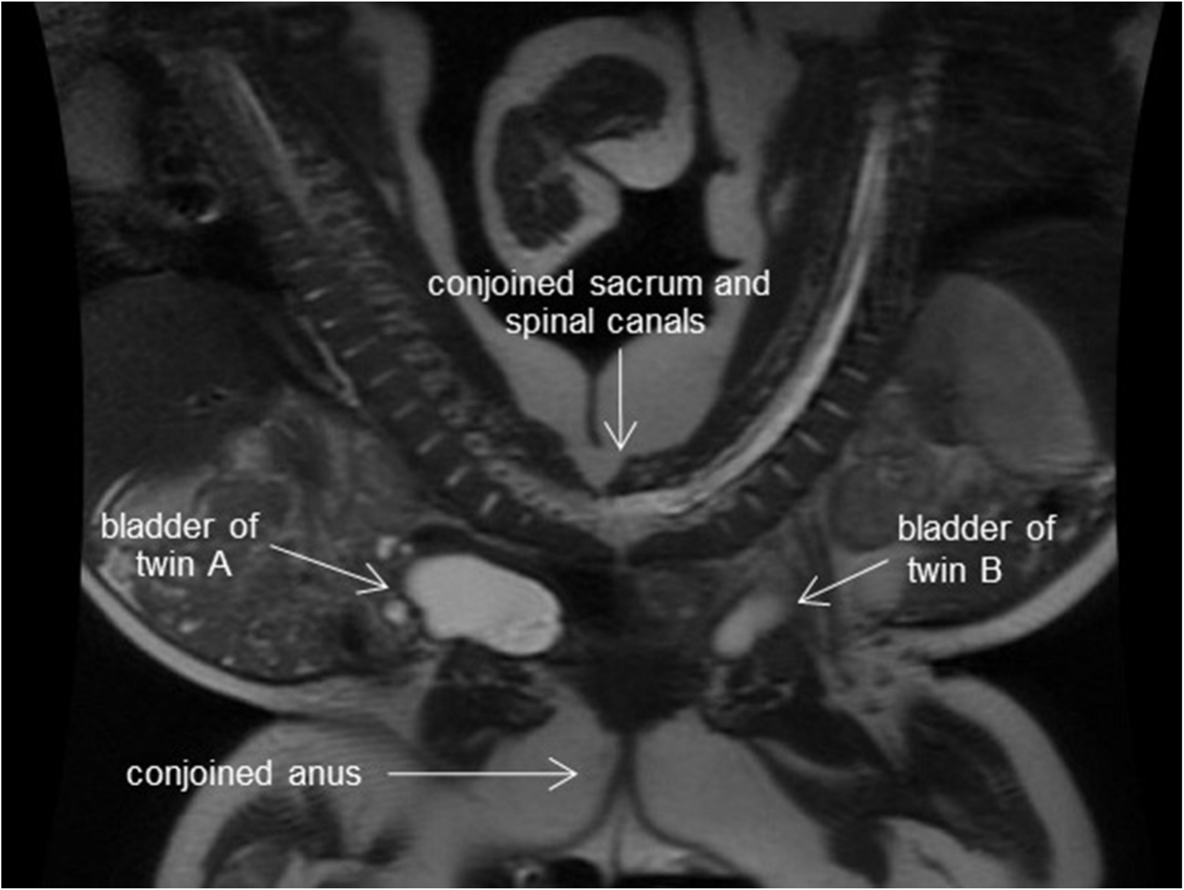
Magnetic resonance imaging of the pygopagus twins; they shared the sacrum, coccyx, anus, and urethra. Each had a separate bladder
